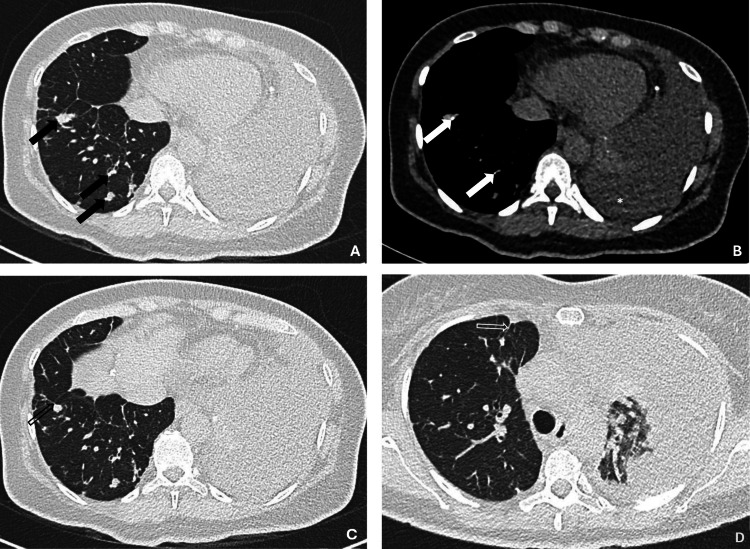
Axial CT images showing complex and mildly spiculated pulmonary nodules (A, black arrows) presenting punctuate calcifications (B, white arrows) and a large left pleural effusion (B, *). Note fissural involvement of pulmonary nodules (C, black open arrow) and the presence of pleural tags (D, white open arrow) CT: computed tomography
